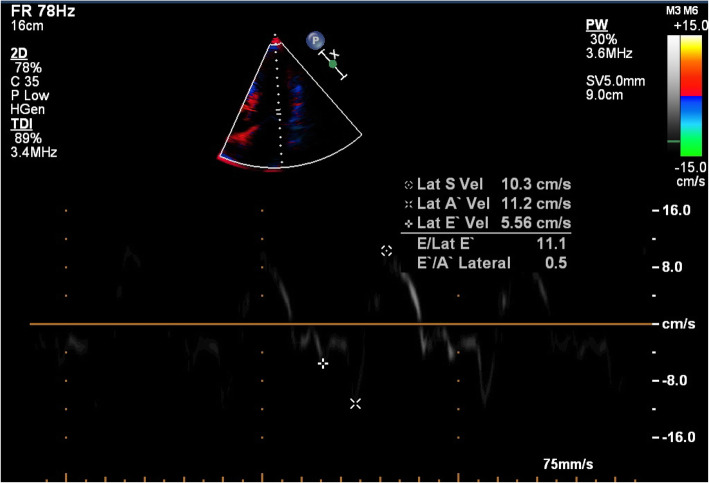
Assessment of diastolic function by lateral mitral annulus tissue Doppler imaging
